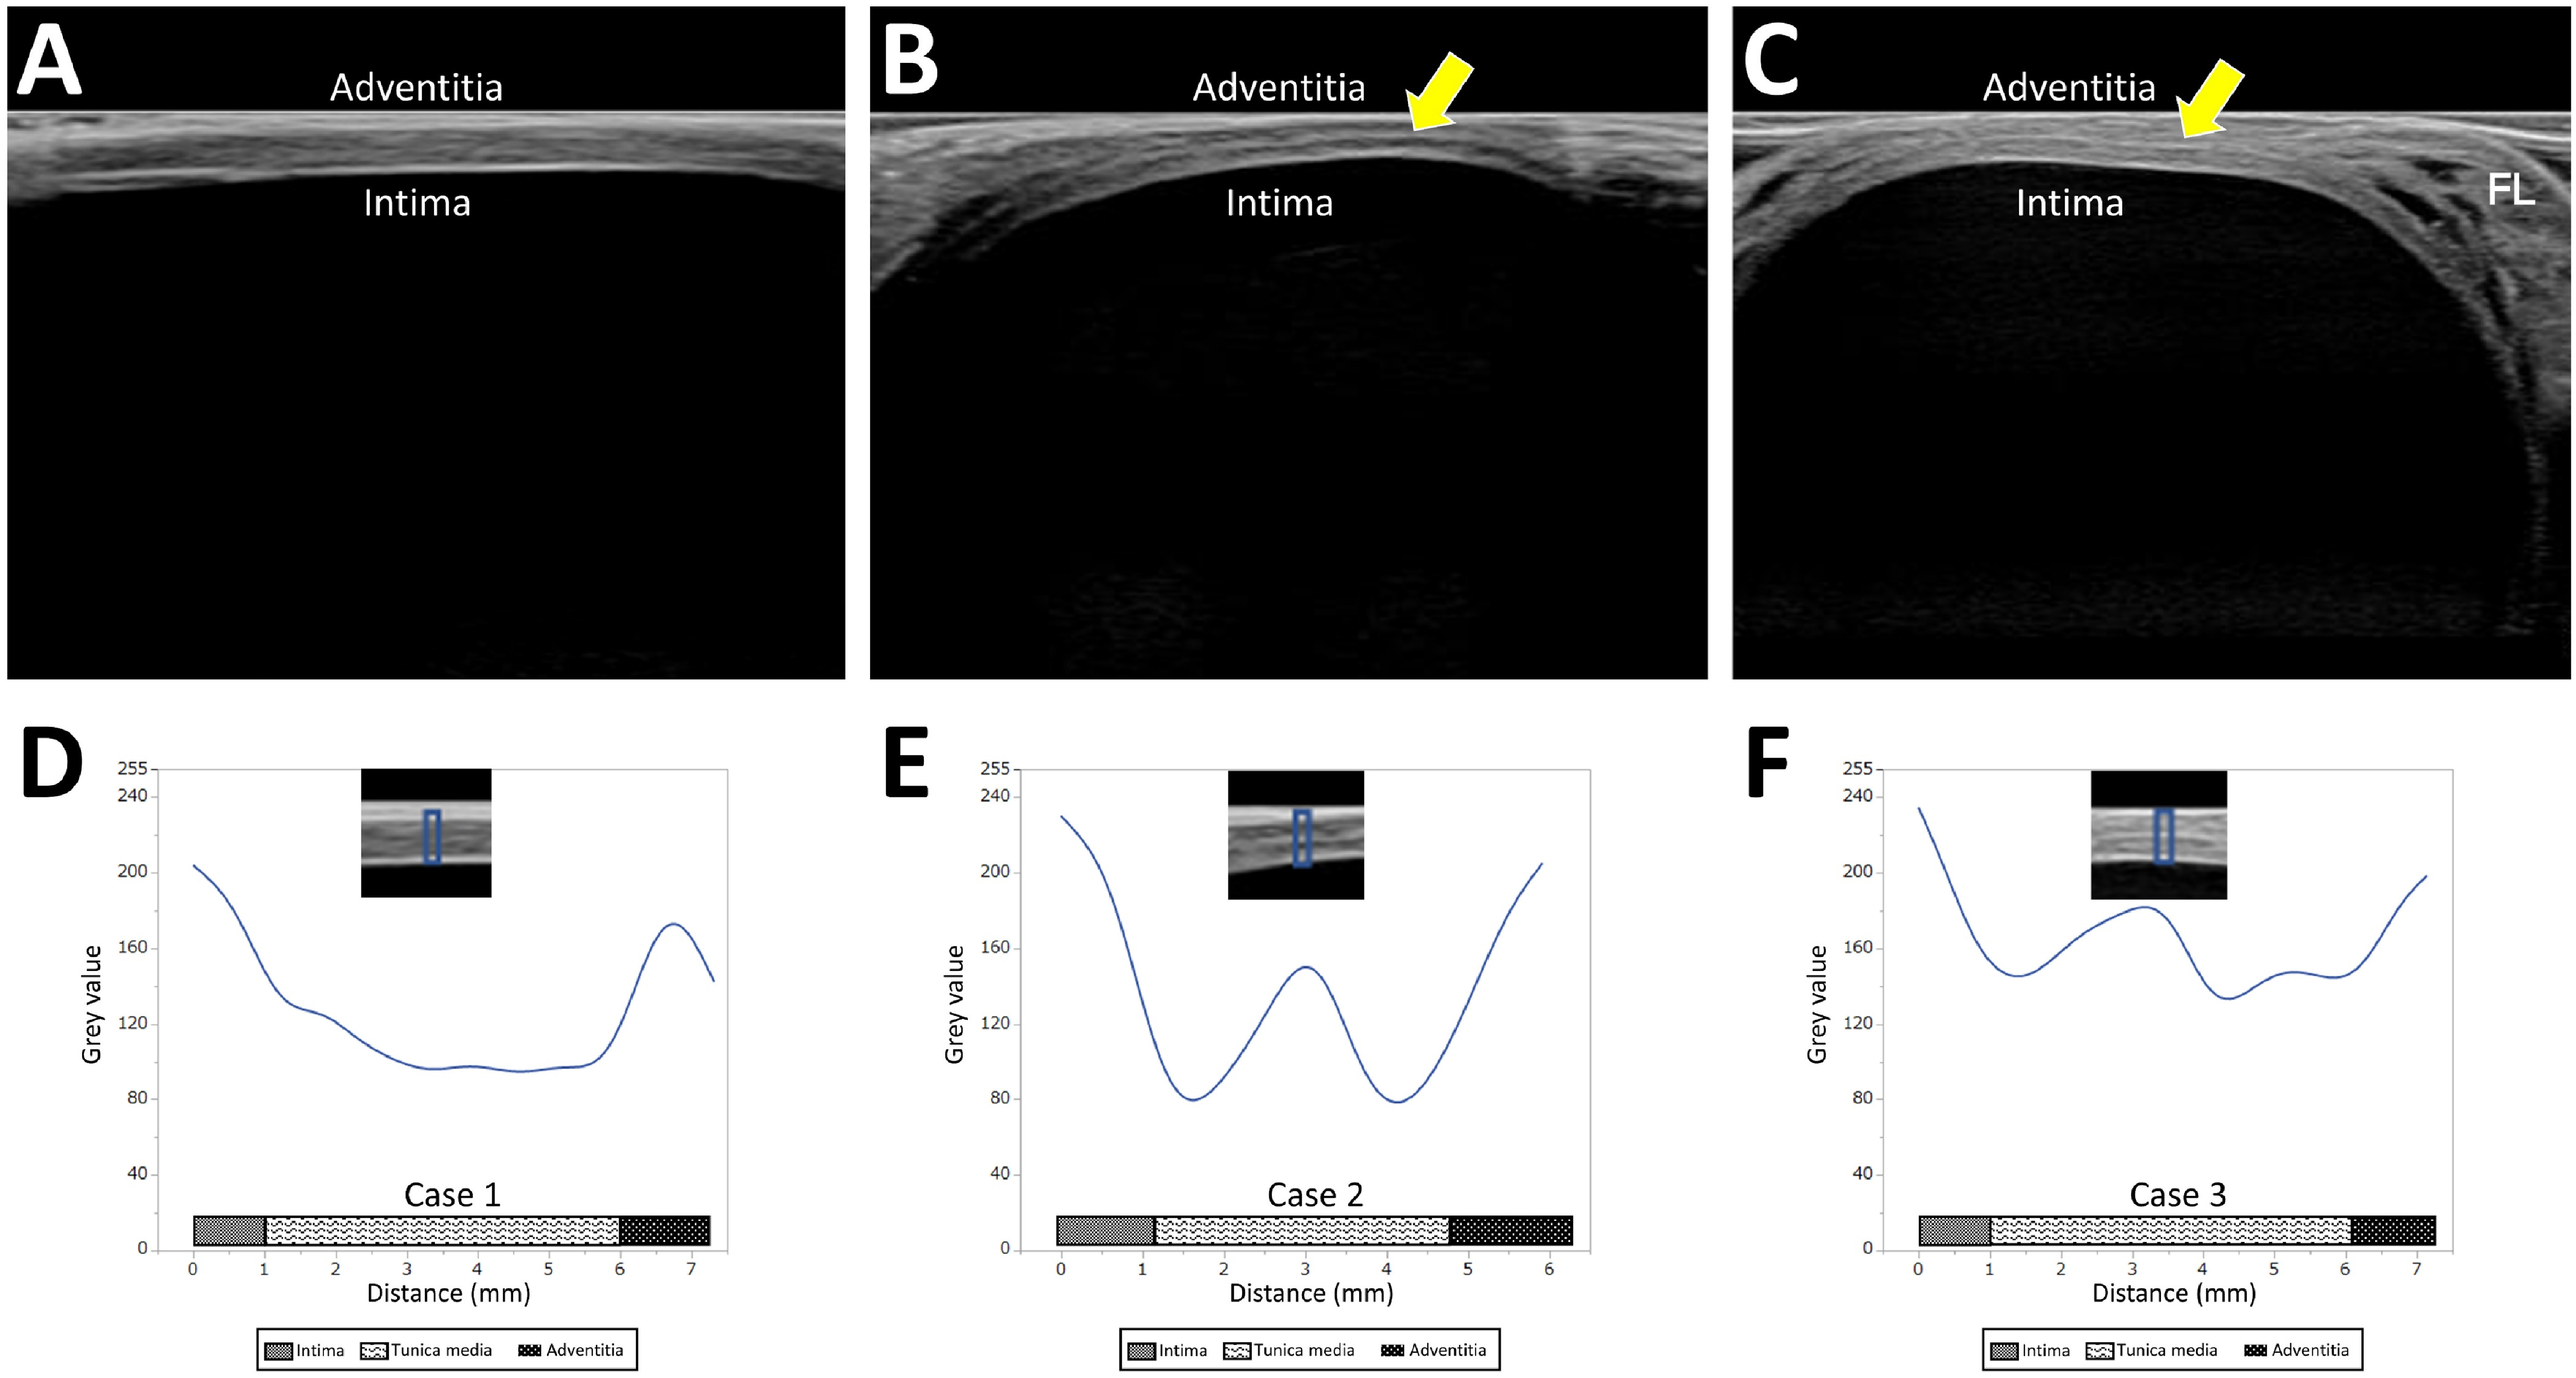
Fig. 1 High-resolution epiaortic ultrasound imaging and line profile of ultrasound intensity of three patients. (A) Case-1 with no aortic pathology. (B) Case-2 with chronic aortic dissection. High-intensity linear changes were observed in the middle of the tunica media (indicated by the yellow arrow). (C) Case-3 with acute type-A aortic dissection. The tunica media was separated according to the line of high intensity (yellow arrow). (D) A line profile of the intensity from the intima to the adventitia (indicated by the blue square) in Case-1. (E) A line profile of the intensity (blue square) in Case-2. (F) A line profile of the intensity (blue square) in Case-3.
